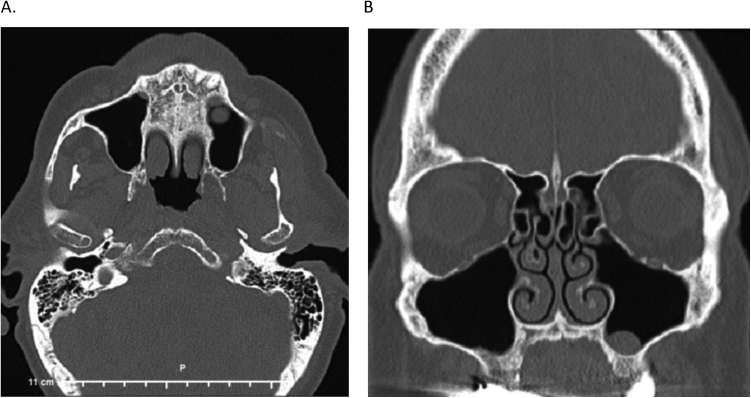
Computed tomography scan of the sinus. Axial view (A) and coronal view (B) of CT sinus scan suggest mild paranasal sinus inflammatory disease and a hyperdense structure within the left maxillary sinus that is likely a small mucous retention cyst or polyp.
